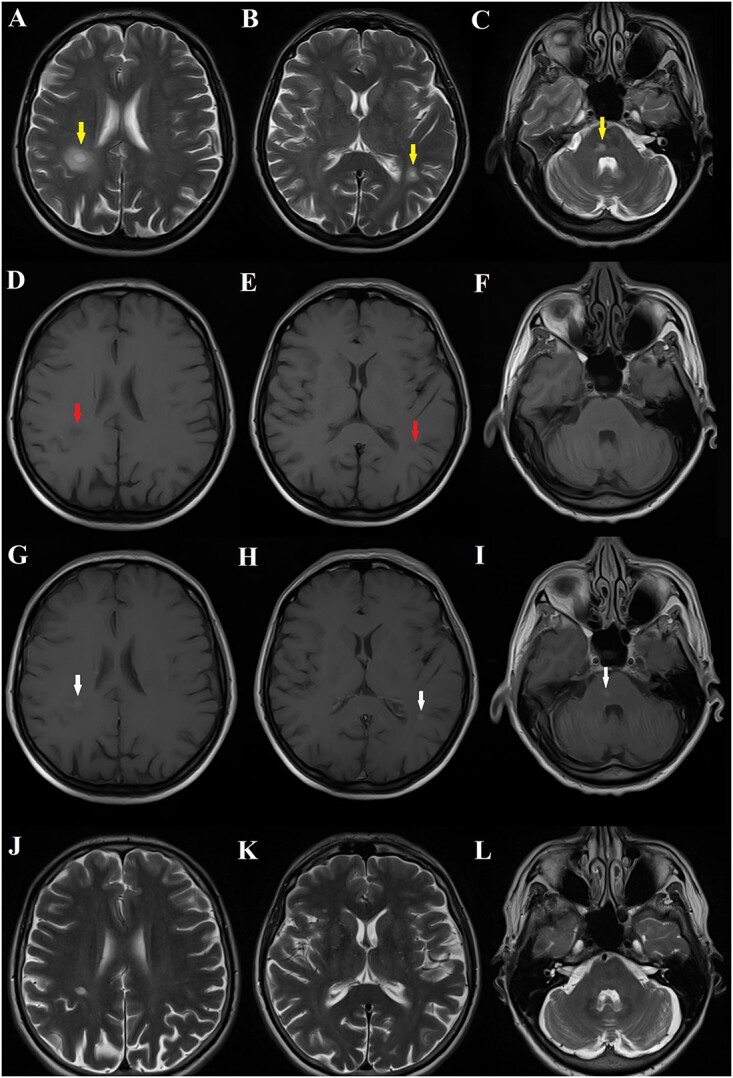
MRI of the brain of a 51-year old woman with a history of tick-borne encephalitis. Axial T2-weighted images (A, B, C) depict high-signal lesions with surrounding edema in the subcortical white matter of the right and left parietal lobe and in the pons. On axial pre-contrast T1-weighted images (D, E, F) two lesions appeared as a low signal intensity and the lesion located in the pons was imperceptible. On axial contrast enhanced T1-weighted images (G, H, I) all lesions showed contrast enhancement. Follow-up MRI demonstrates no new lesions and that previously demonstrated lesions markedly reduced in size, with some residual abnormal T2 signal in the cerebral hemispheres (J, K, L).
